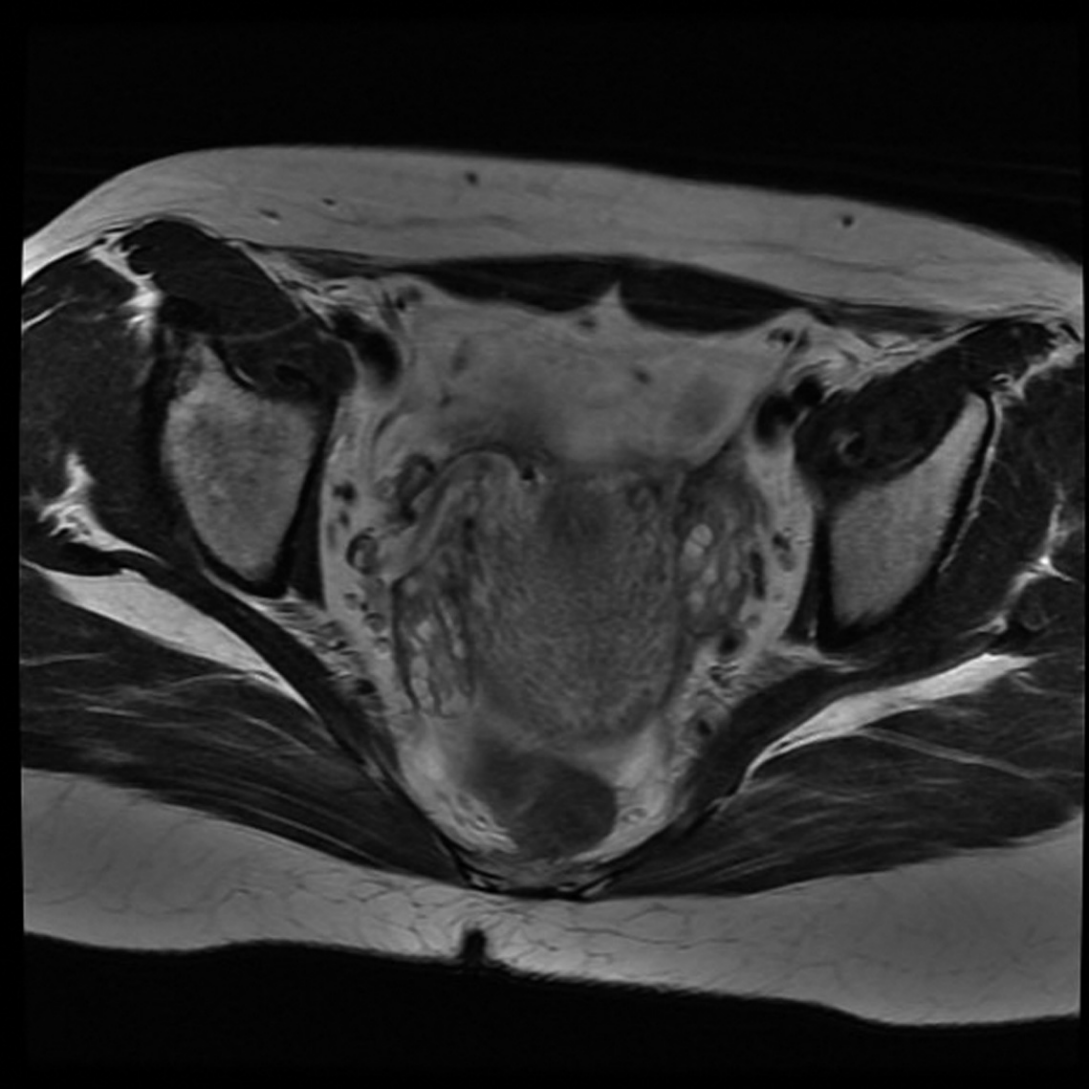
MRI scan showing gravid uterus and bilateral cystic ovaries.
